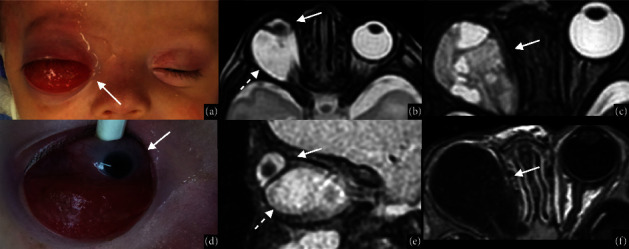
Coloboma/microphthalmia with cyst vs congenital cystic eye. A thirteen-day-old girl with suspected right anophthalmia with cyst (white arrow in (a)) underwent an MRI whose axial (b) and sagittal (e) T2WI sequences showed the presence of severe microphthalmia (white arrows in (b, e)) with associated cyst (dotted white arrow in (b, e)), that was subsequently confirmed during clinical observation under sedation (d). Axial high-resolution T2WI (c) and axial postcontrast T1 (f) in a two-month-old boy affected by congenital cystic eye show a multilobulated cystic formation without an ocular globe (white arrow in (c, f)).
